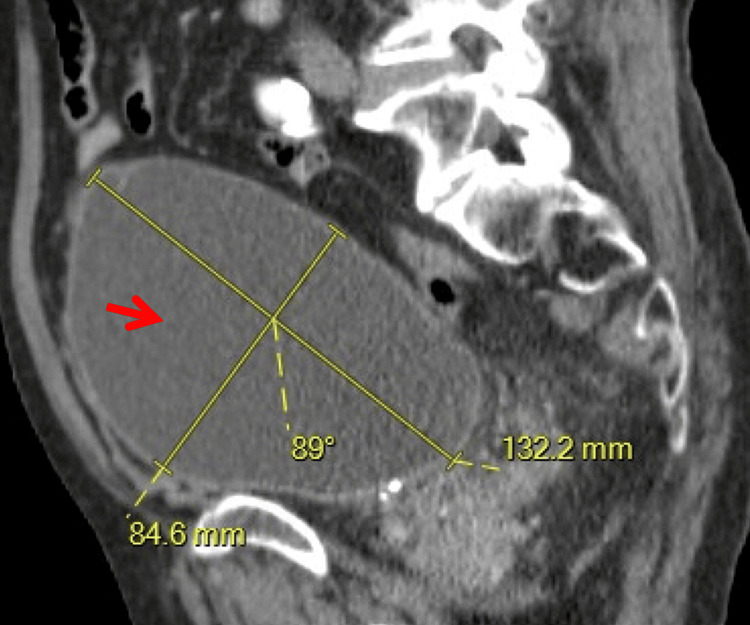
CT abdomen and pelvis, sagittal view. Severely distended urinary bladder (arrow) extending superiorly within the pelvis, consistent with acute urinary retention
